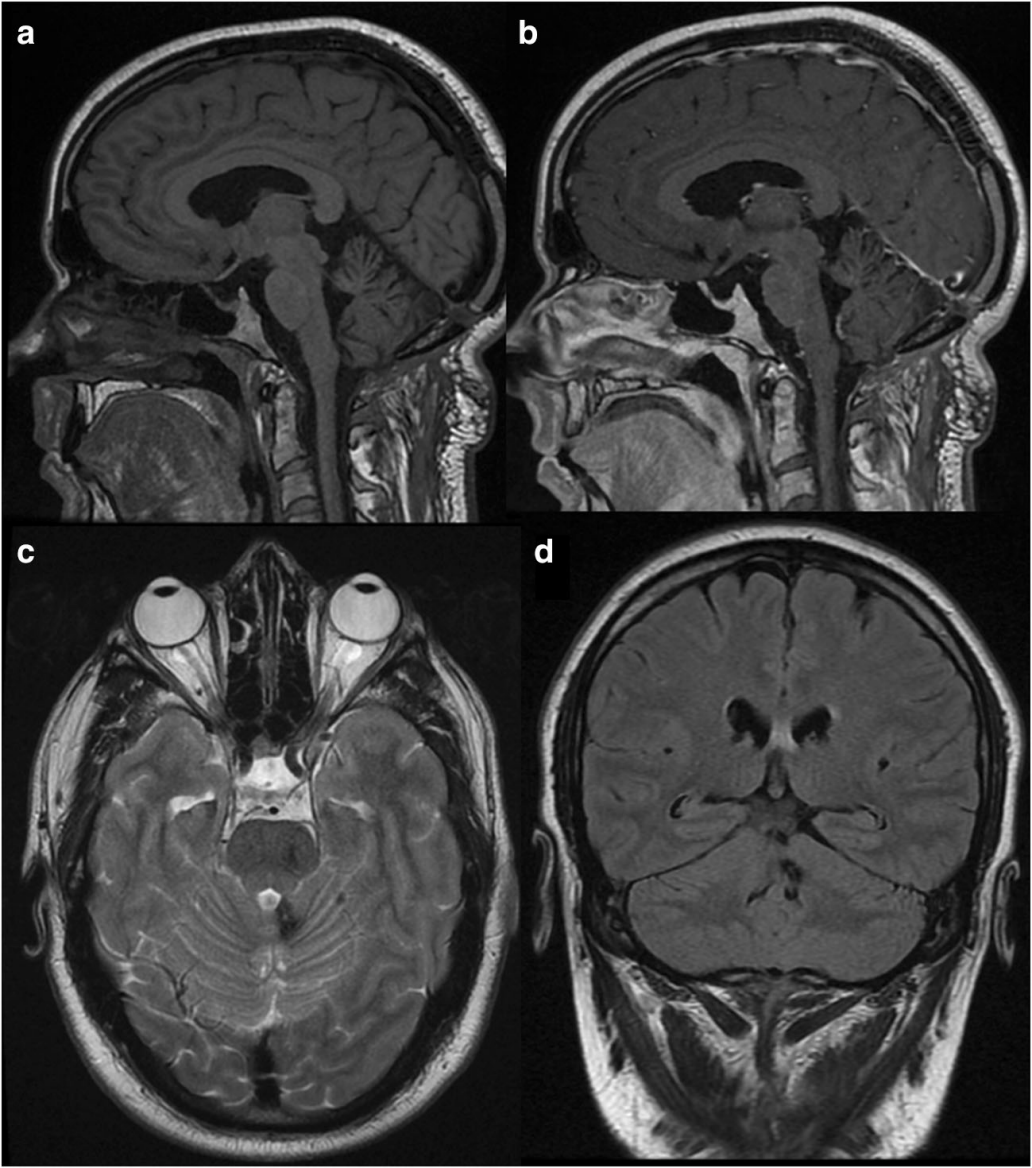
Post-operative MRI: T1-weighted images—non-contrast (a) and with gadolinium (b)—document absence of residual disease or complications related with the surgical resection. T2 weighted (c) and FLAIR (d) show the residual haemosiderin ring collapsed into the surgical cavity
